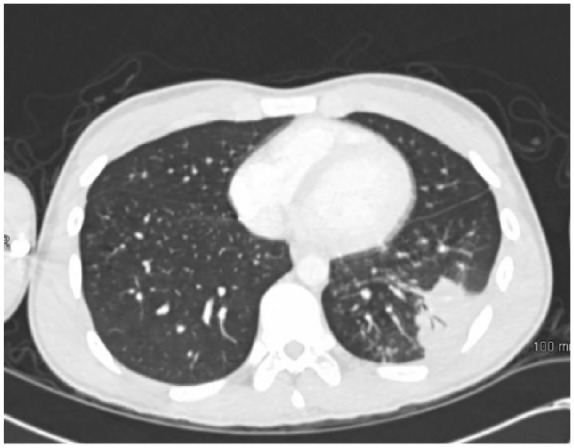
Chest computed tomography on admission for case 1.
